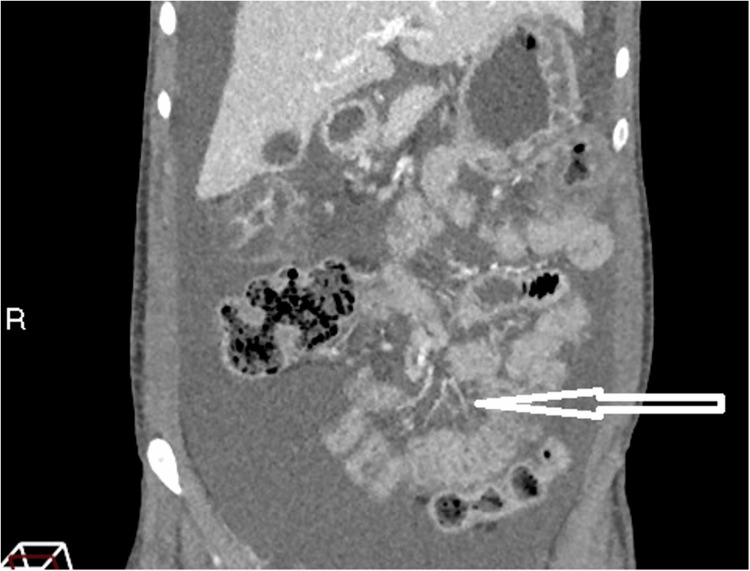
Contrast-enhanced CT of the abdomen and pelvis in the coronal plane showing the “comb sign” The arrow points to the mesenteric vessels. These vessels are abnormally enlarged due to reactive hypervascular filling with blood. This is a response to active inflammation. The appearance of the engorged vessels interspersed with fat (or large volume ascites, as seen in this figure) resembles the appearance of a hair comb. This sign helps differentiate active inflammatory disease from other causes such as metastatic deposits or bowel lymphoma, which appear hypovascular.
